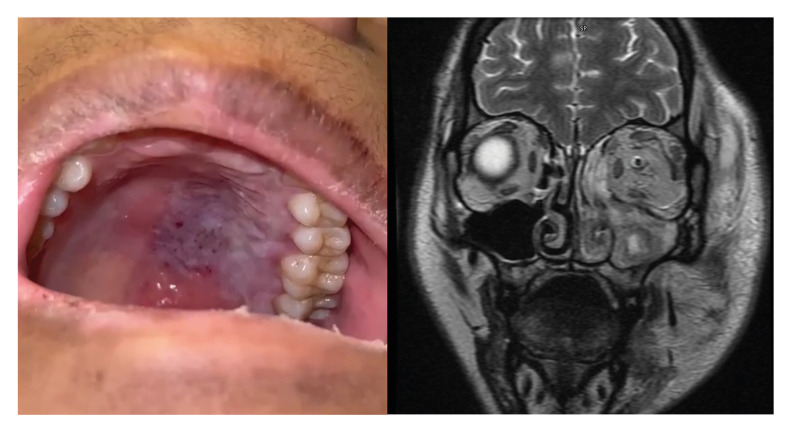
(Left) Purplish mucous lesion of left hemipalate with necrosis; (Right) Coronal section of MRI demonstrating involvement of mucormycosis infection of left ethmoid sinus, lateral wall of nose, maxillary sinus, floor and medial wall of the left orbit.
